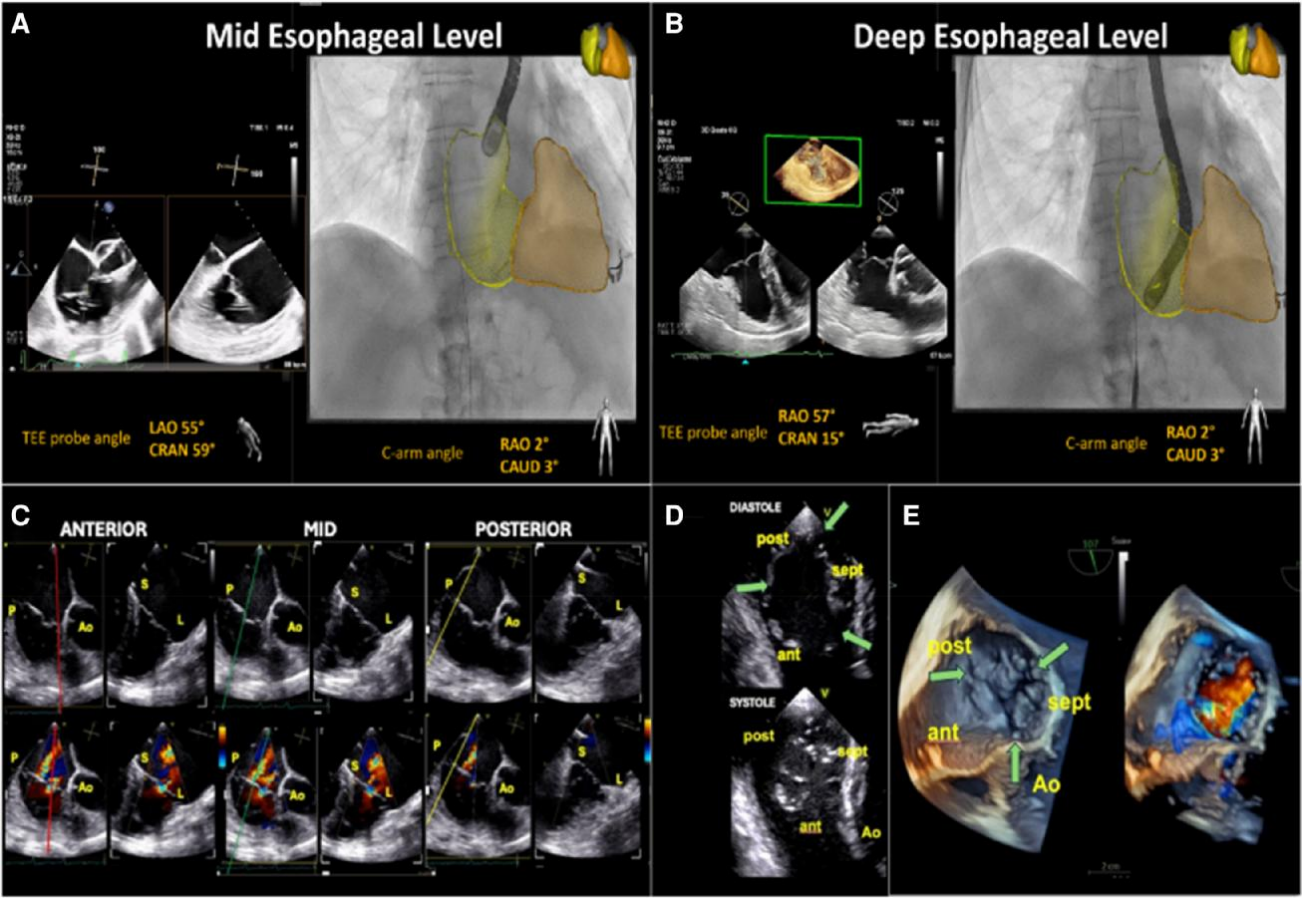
Key transoesophageal echocardiographic views for tricuspid valve assessment. (*A*) RV inflow–outflow view with simultaneous orthogonal views (Xplane, Biplane) depicting the RV inflow (right side focused four chamber view), acquired using a three-dimensional TEE probe at the mid-oesophageal level with the corresponding C-arm angulation and its superimposed projection on the angiography. (*B*) RV inflow view (right side focused four chamber view) with simultaneous orthogonal views (Xplane, Biplane) depicting the RV inflow–outflow view, acquired using a three-dimensional TEE probe at the deep-oesophageal level with the corresponding C-arm angulation and its superimposed projection on the angiography. (*C*) At the mid-oesophageal level, the three panels represent progressive slices of the tricuspid valve from anterior (red line, left panel), central (green line, middle panel), to posterior (yellow line, right panel), with the latter being the most distant from the aortic valve and therefore, posterior. Colour Doppler imaging in each panel enables comprehensive functional assessment, including leaflet motion, leaflet tethering, leaflet length, and identification of the primary origin of tricuspid regurgitant flow. AO, aortic valve (anterior); L, lateral; P, posterior; S, septal. (*D*) Short-axis transgastric view and 3D en-face view of the tricuspid valve. Left panel: Short-axis view of the tricuspid valve obtained from the transgastric level. The image depicts the tricuspid valve in diastole with three leaflets and three commissures indicated by green arrows (upper panel), alongside the same valve in systole (lower panel). Ant, anterior; AO, aortic valve (anterior); Post, posterior; Sept, septal. (*E*) Three-dimensional en-face TEE view from the right atrial perspective, oriented similarly to the transgastric short-axis view, with the aortic valve positioned at 5 o’clock on a clock-face orientation. The three leaflets and corresponding commissures (green arrows) are clearly visible. The right panel demonstrates the same 3D view with colour Doppler imaging, highlighting the central origin of tricuspid regurgitant flow between the coaptation surfaces of the anterior and septal leaflets. Ant, anterior; AO, aortic valve (anterior); Post, posterior; Sept, septal.
